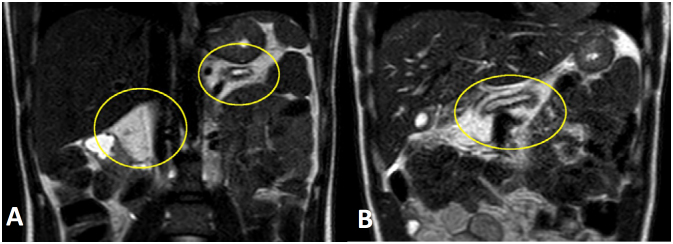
(A and B) T2-weighted axial images without fat signal saturation. The pancreatic parenchyma is replaced by adipose tissue.
